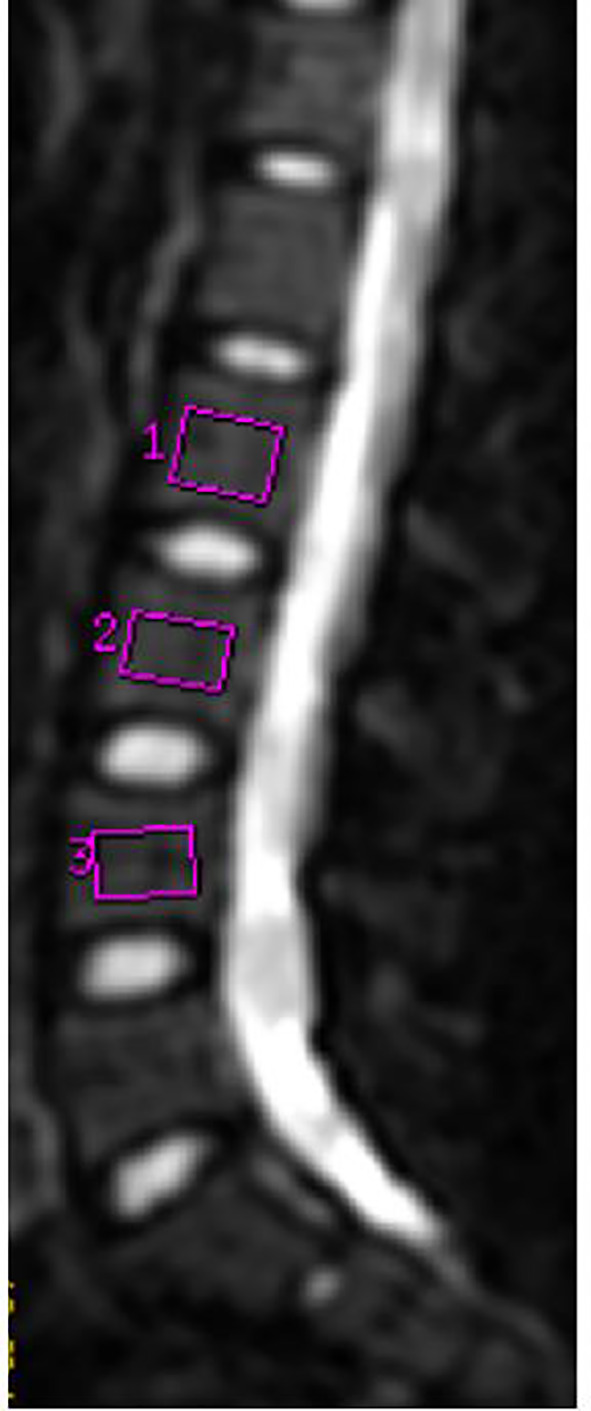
MR image in a 24-year-old woman. On the median sagittal image with b = 0, L2–L4 vertebrae were selected to delineate ROI.
